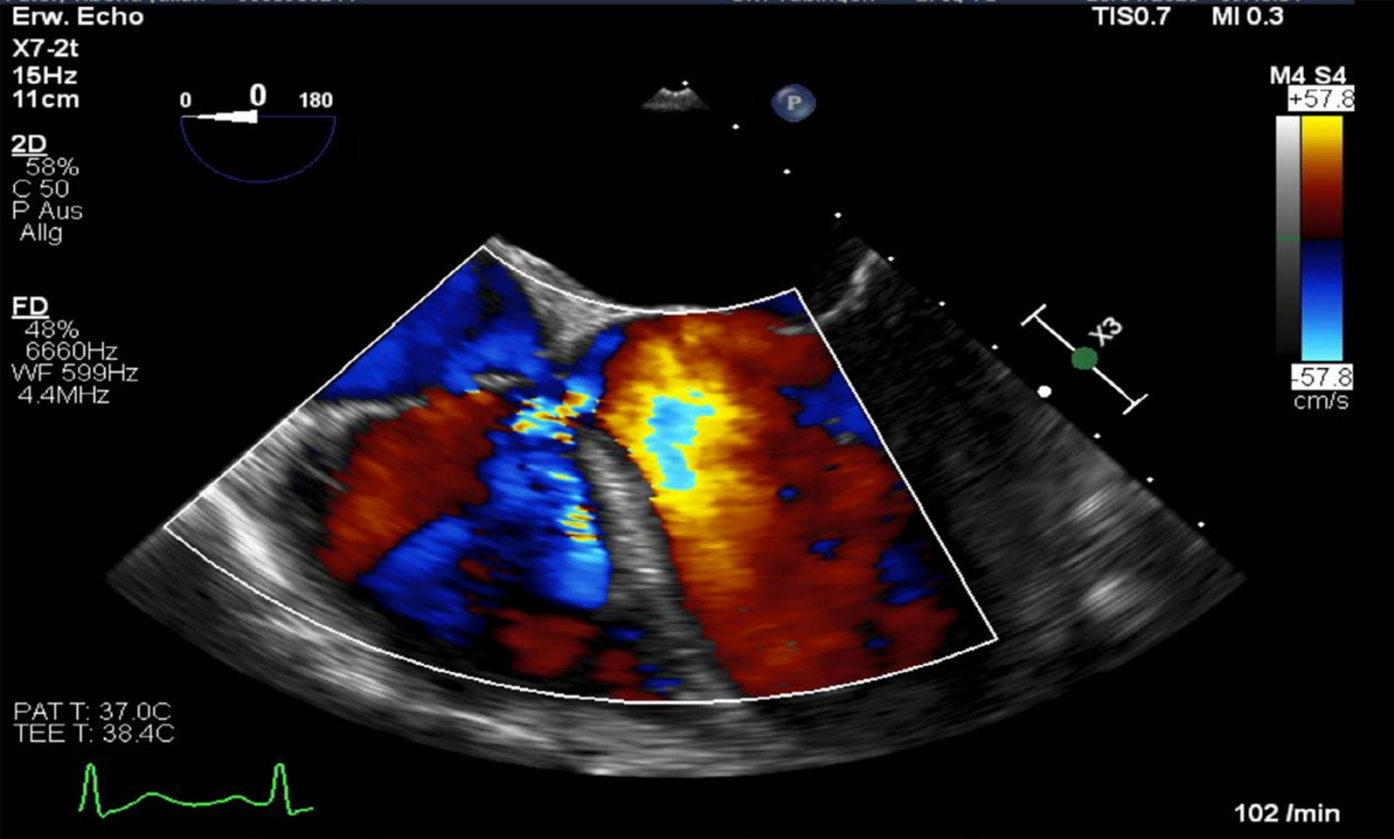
Transoesophageal echocardiography showing a left-to-right shunt in colour Doppler corresponding to a VSD
